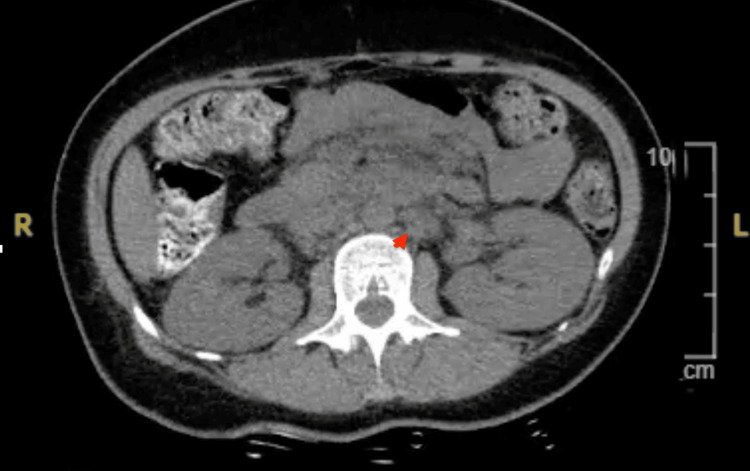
CT of the abdomen and pelvis with significant retroperitoneal lymphadenopathy in case 2
